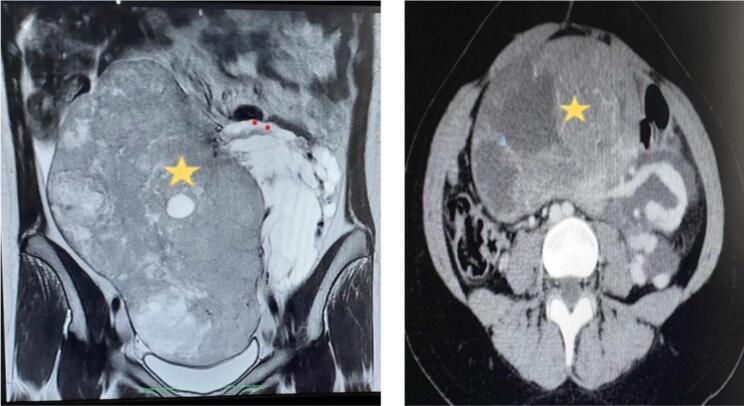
Abdominopelvic MRI. (star) A large, oblong, right paramedian, solid-cystic abdominopelvic mass with predominant solid areas showing mild hyperintensity on T2, hypointensity on T1, hyperintensity on diffusion with restricted diffusion, and enhancement after gadolinium injection.
